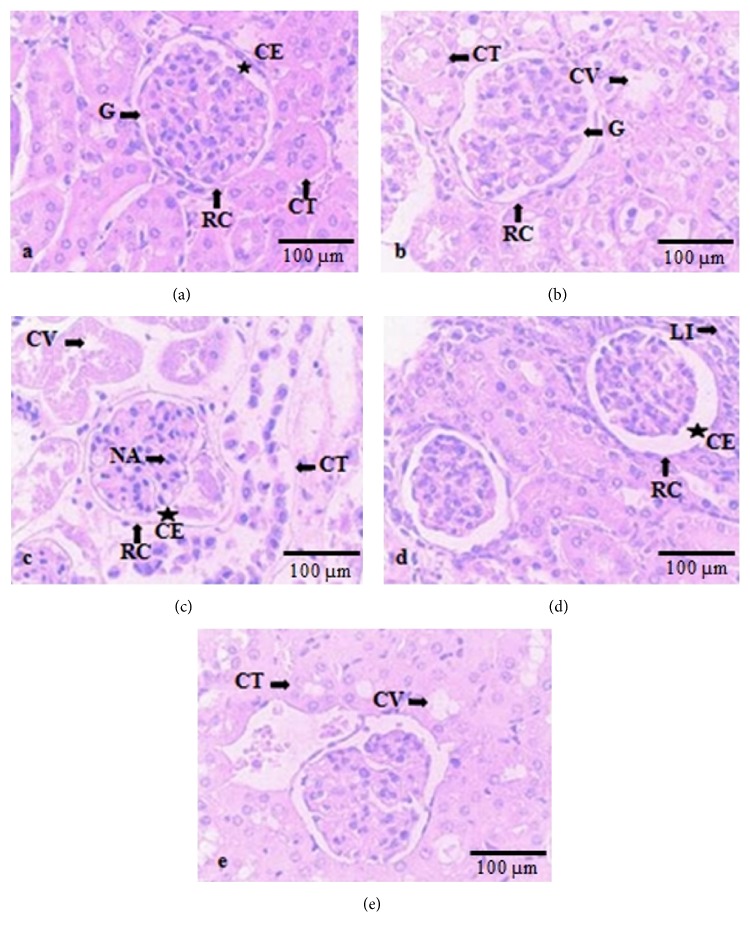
Kidney sections of (a) control, (b) UA-micro (2000 mg/kg; p.o.), (c) UA (2000 mg/kg; p.o.), (d) UA (300 mg/kg; p.o.), and (e) UA (50 mg/kg; p.o.). RC: renal corpuscle; CS: capsular space; LI: leukocytes infiltrate; CT: convoluted tubules; G: glomerulus; CV: cytoplasmic vacuolation; NA: nuclear acidophilia (×400 magnification).
